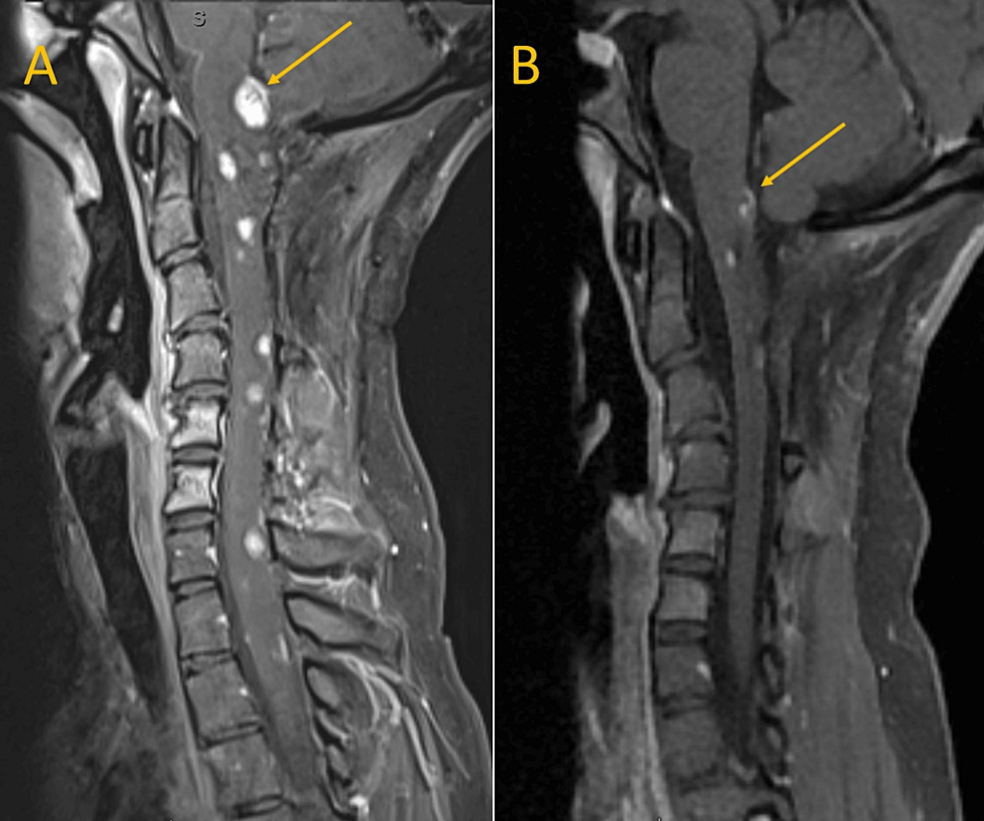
Comparison of patient's initial magnetic resonance imaging (MRI) of the cervical spine versus at 18-month follow-up (T1 post-contrast) (A) Surveillance MRI showing multiple enhancing lesions throughout the neuraxis with the largest lesion at the cervicomedullary junction measuring 9 x 9 x 12 mm (yellow arrow). (B) An 18-month follow-up MRI post the belzutifan treatment showing a durable clinical response (yellow arrow).
